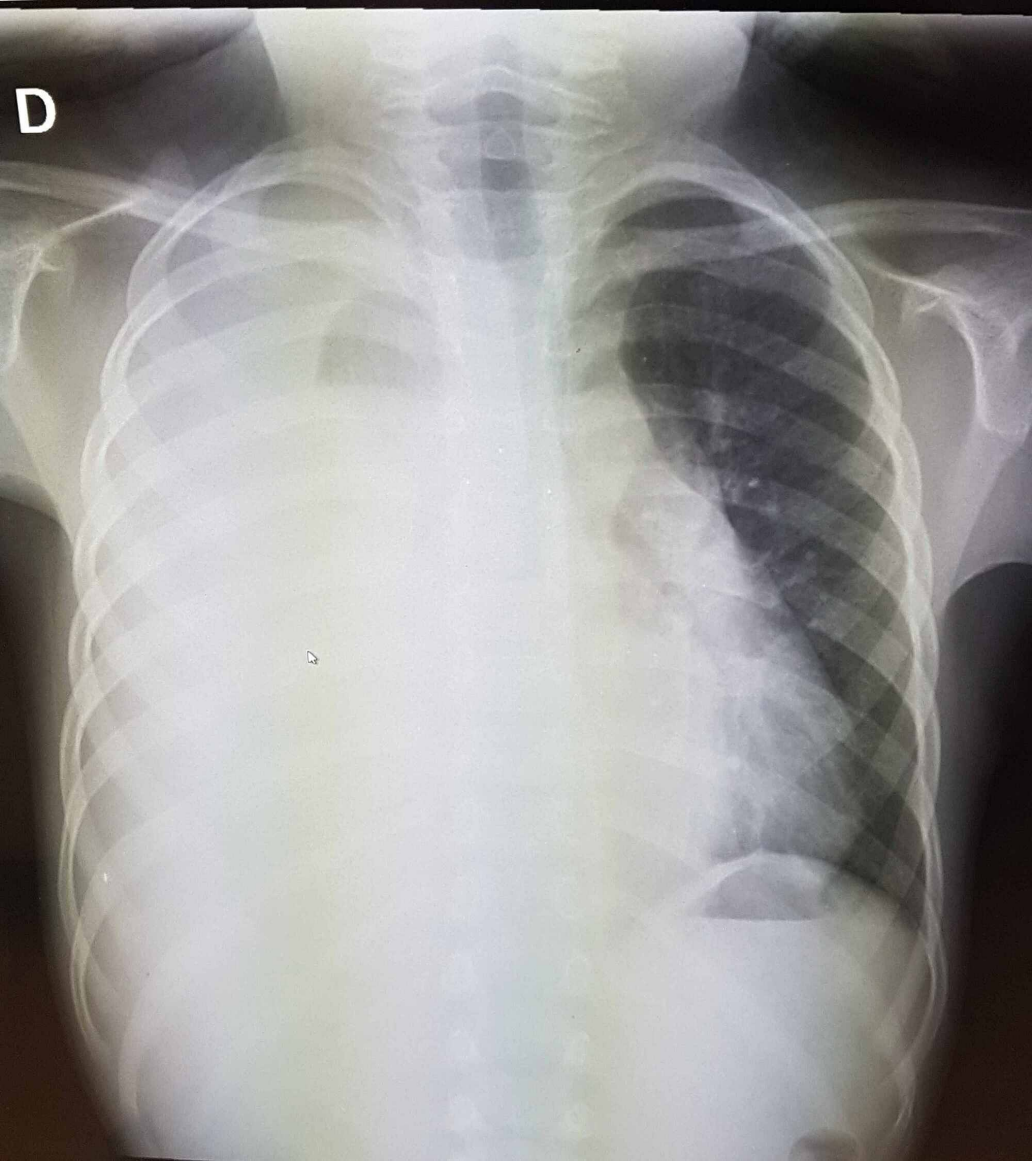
Standard chest x-ray showing massive right pleurisy
